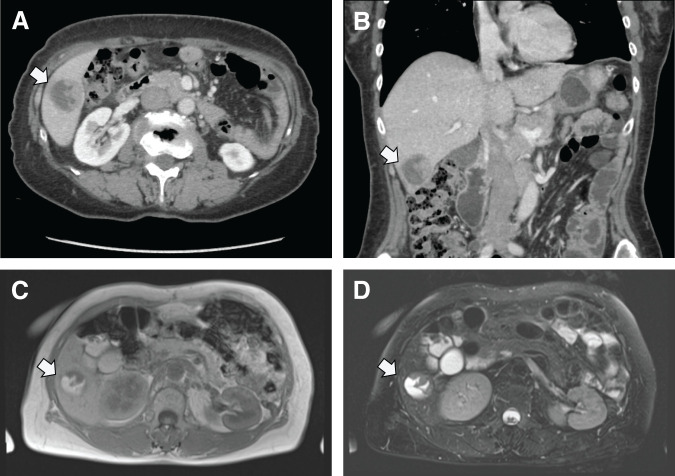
(A, B) Abdominal CT findings of a 3.4-cm solid mass in the 6th liver segment. The tumor was well defined and rounded, with heterogeneous internal enhancement after contrast injection. (C, D) On MRI, the tumor exhibited signal characteristics similar muscle on T1-weighted images and a higher signal than muscle on T2-weighted images. In addition, it showed fluid characteristics of cysts with high water content.
